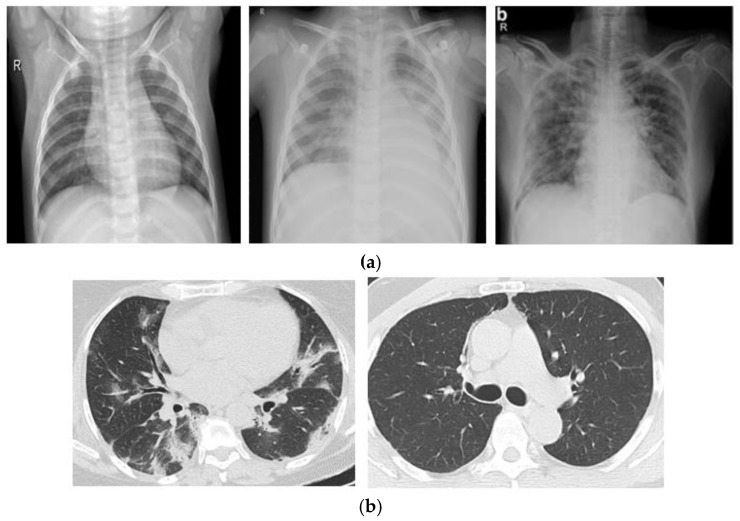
(a) X-ray Radiographs of normal, pneumonia and COVID patients. (b) CT Scan images of COVID and normal patients.
